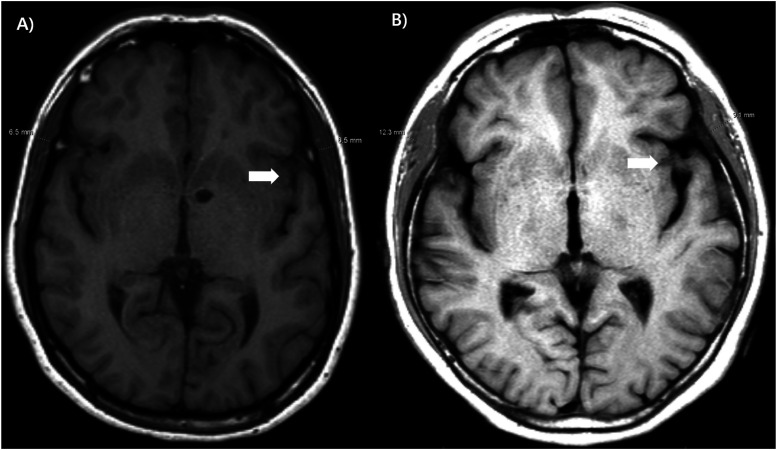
Temporalis muscle thickness (TMT) measurement. TMT was measured perpendicular to the long axis of the temporalis muscle on axial T1-weighted images using the Sylvian fissure (white arrow) as anatomical landmarks. Bilateral TMTs were measured. (a) Axial T1-weighted image from a non-ambulatory adult with cerebral palsy, (b) Axial T1-weighted image from an ambulatory adult with cerebral palsy.
